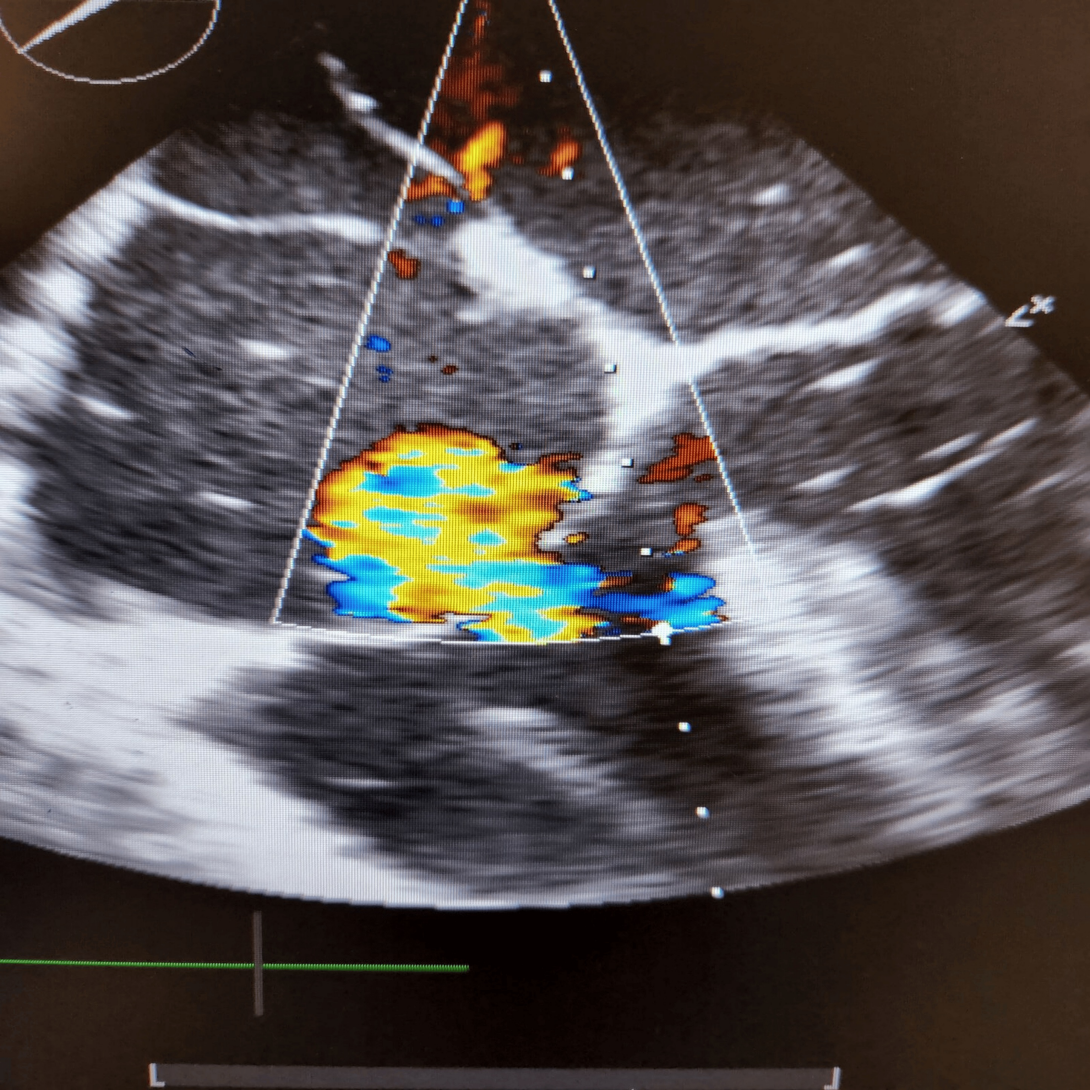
Gas bubbles seen in both the atria (with patent foramen ovale) in a 2D echo (echocardiogram)
